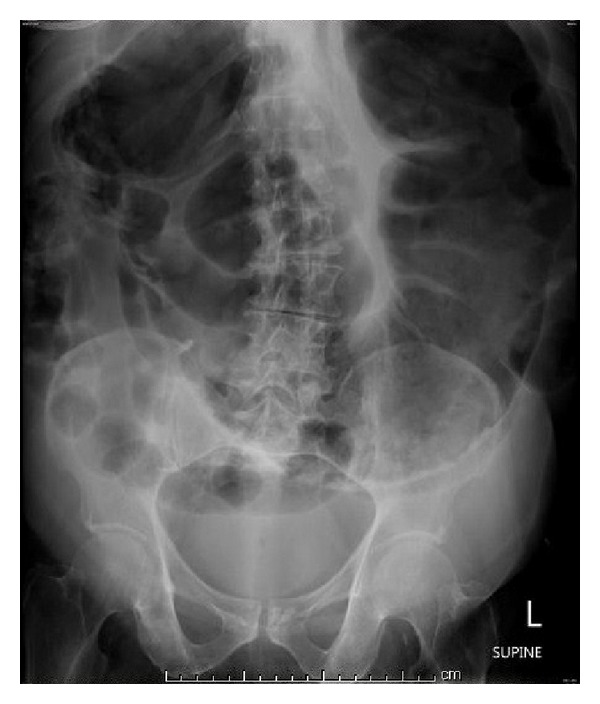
A plain abdominal X-ray showing the colonic dilatation with a cutoff at the rectum.
